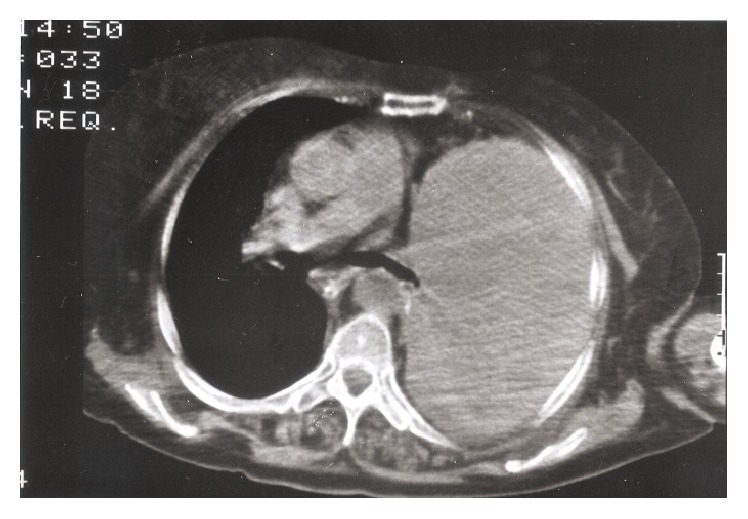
Computed tomography of the thorax showing a large mass in the left hemithorax.
